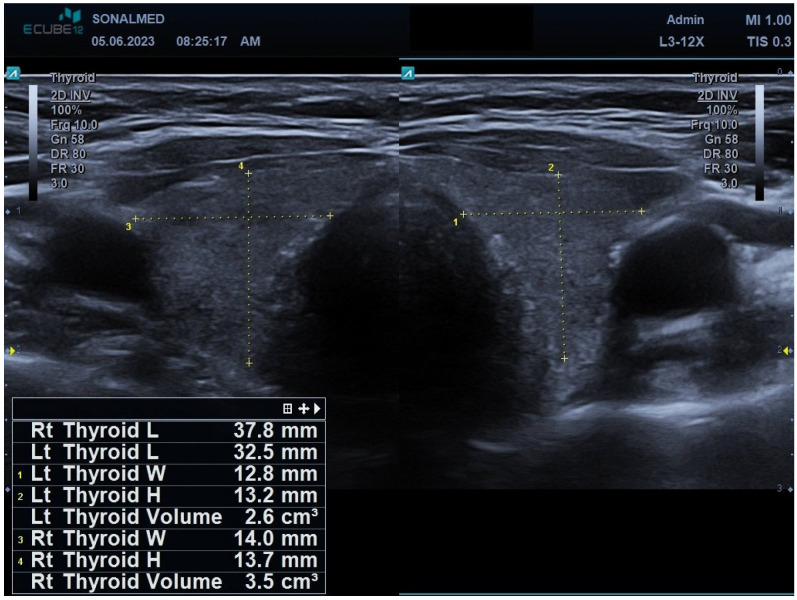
Transverse grey-scale ultrasound image of the thyroid gland after eight cycles of sunitinib therapy. The image demonstrates marked reduction in thyroid volume (left lobe: 2.6 cm^3^; right lobe: 3.5 cm^3^), accompanied by increased parenchymal heterogeneity and decreased echogenicity. These findings are consistent with progressive thyroid atrophy and structural deterioration associated with ongoing sunitinib treatment. Note: Yellow dotted lines—measurement markers delineating the thyroid boundaries for volumetric assessment of the gland.
